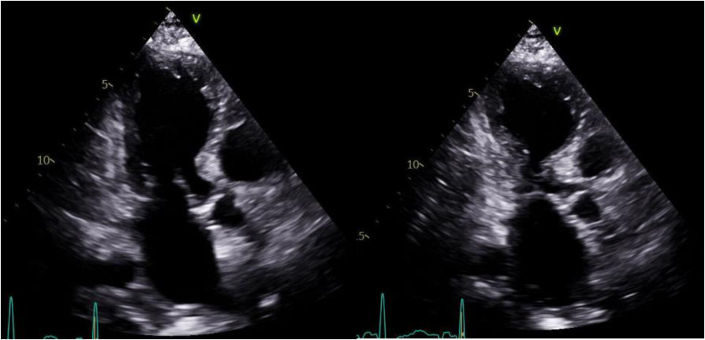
Transthoracic Echocardiogram (Left) Apical 3-chamber view showing diastole) compared with (right) systolic apical ballooning of the left ventricle.
